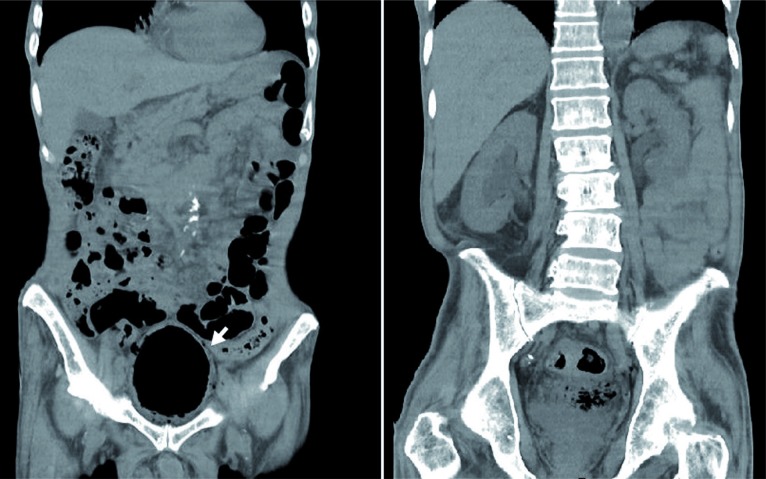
Abdominal computed tomography revealed bladder tamponade with massive air accumulation in bladder lumen and bladder wall (arrow), which leaded to bilateral hydronephrosis.
